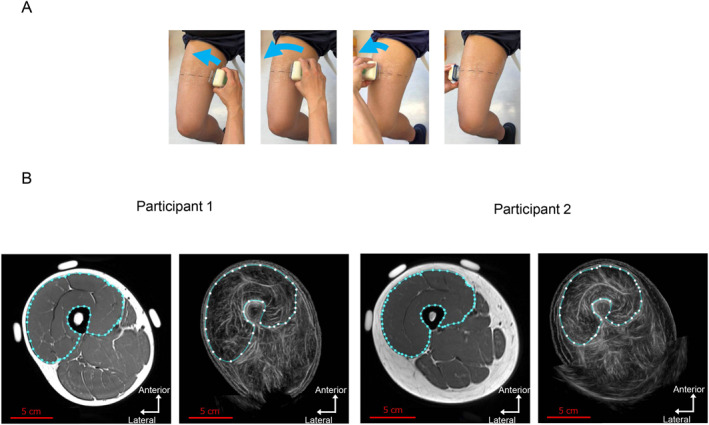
Measurement of the quadriceps femoris muscle cross‐sectional area (CSA). (A) Experimental setup demonstrating the use of the extended field‐of‐view wireless palm‐sized ultrasound device. (B) Comparison of representative CSA images from magnetic resonance imaging (left) and ultrasonography (right) from two participants. Participant 1 was a male (age: 23 years; height: 1.79 m; body mass: 67.3 kg), and Participant 2 was a female (age: 23 years; height: 1.59 m; body mass: 54.2 kg).
